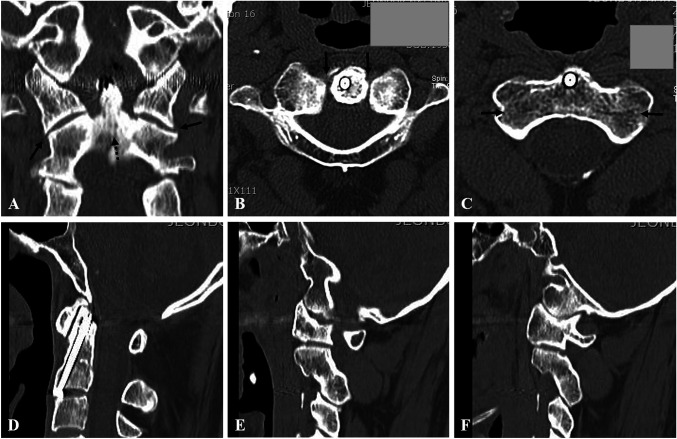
At 2 years after surgery, follow-up coronal (A) CT scan showing fusion of the type 3 dens fracture (dotted dark arrow) and good reduction of C1-2 lateral subluxation (dark arrows). Axial (B and C) CT scans showing symmetry of the C1-2 joints (dark arrows) and union of the fractures of both C2 lateral masses (dark arrows). Sagittal (D) CT scan showing fusion of a type 3 dens fracture with correction of angulation. Right and left parasagittal (E and F) CT scans showing fusion of fractures of both pars interarticularis, a type 1 hangman fracture.
